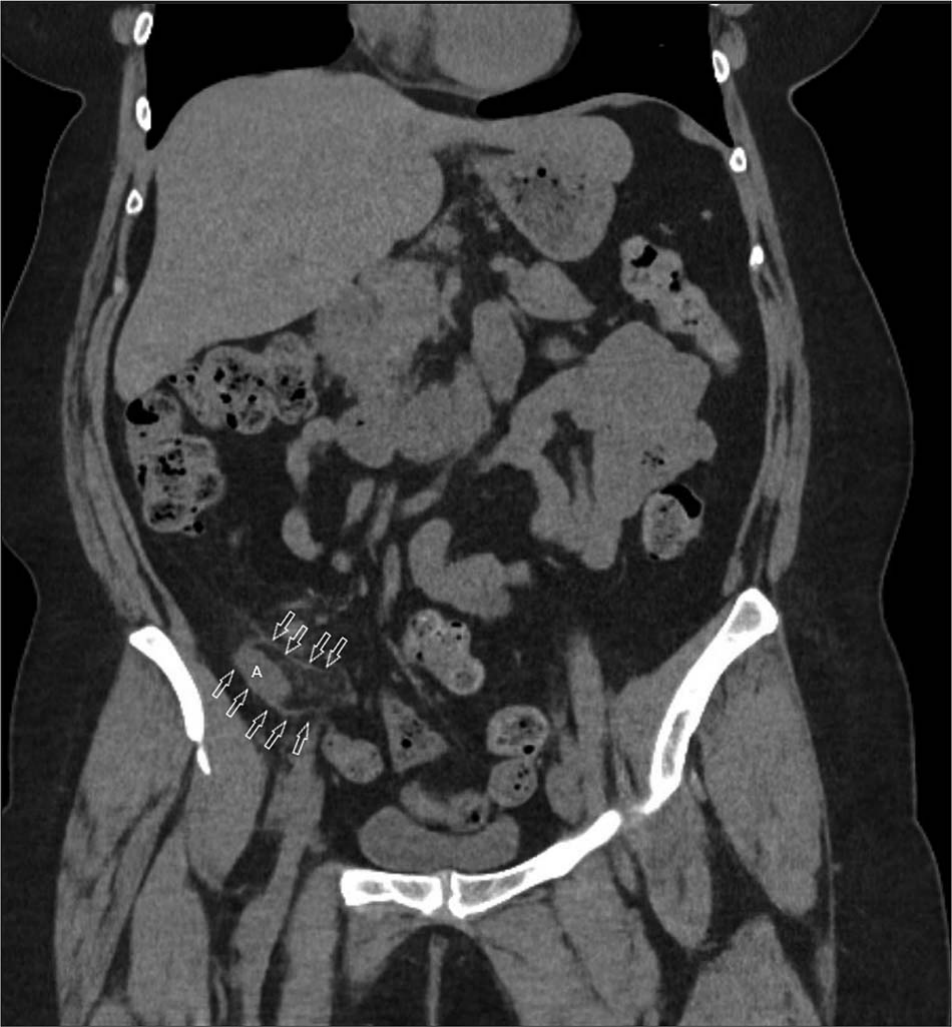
Coronal image CT scan with IV contrast with arrows denoting appendix in proximity to iliacus space. (A) Appendix.
